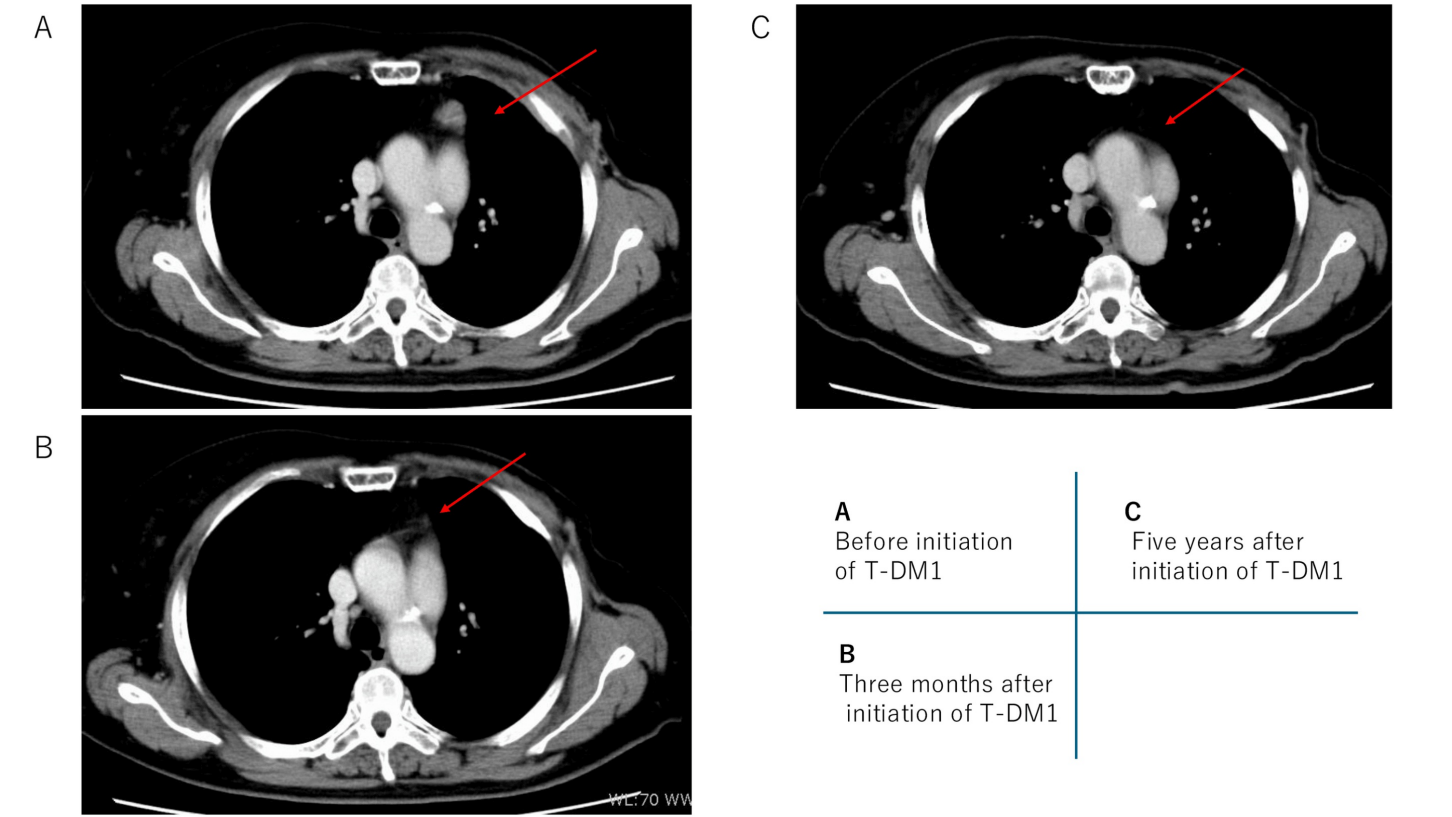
Serial follow-up computed tomography images demonstrating sustained complete response of mediastinal lymph nodes following ado-trastuzumab emtansine (T-DM1) therapy (A) CT image obtained prior to initiation of T-DM1, showing enlarged mediastinal lymph nodes (red arrow). (B) CT image obtained three months after initiation of T-DM1, demonstrating complete disappearance of the mediastinal lymph nodes, consistent with a complete response. (C) CT image obtained five years after initiation of T-DM1, showing sustained complete response with no evidence of recurrent mediastinal lymphadenopathy.
